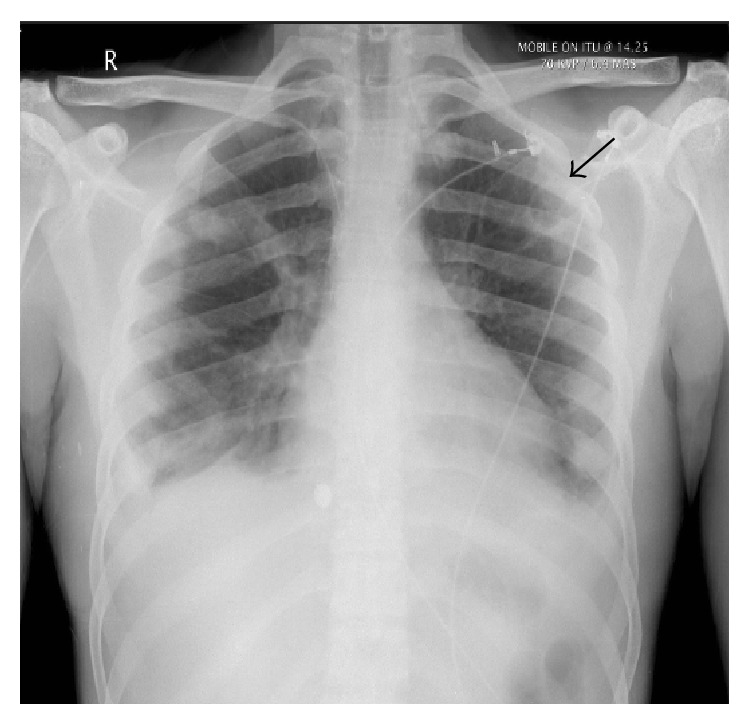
Chest X-ray on admission: arrow points to cavitating lesion in the upper zone of the left lung. Bilateral pleural effusions are evident.
